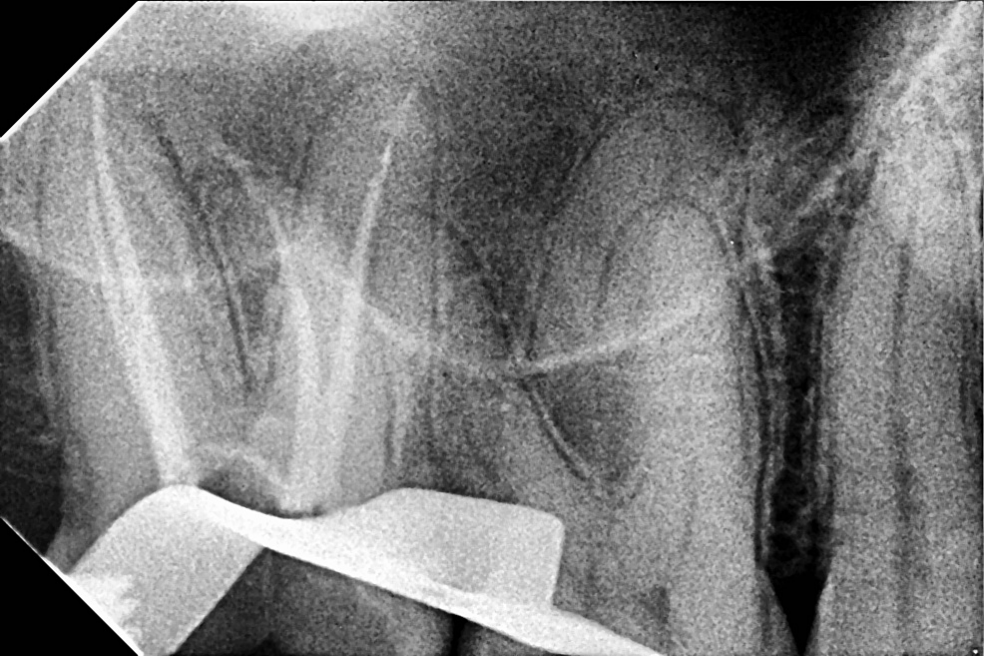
Obturation radiograph of the upper right second molar
